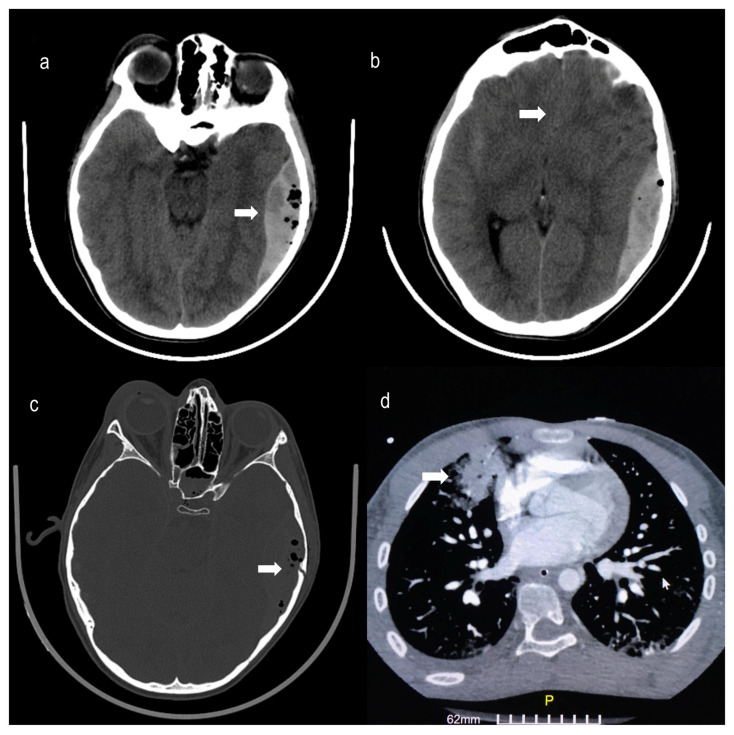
Brain Computed Tomography (CT) scan within 2 h of the accidentshowing: (a) acute left temporal and parietal epidural hematoma (arrow) (5 × 8 cm) with (b) mass effect and 4 mm midline shift (arrow), (c) Bone window of head CT scan showing a complex nondisplaced comminuted fracture of the left temporal bone (arrow), and (d) Chest CT showing contusions of the right upper lobe (arrow), right middle lobe and lower left lobe of the lungs (GE Healthcare Revolution 128-slice CT scanner, USA—Slice thickness of 1.25–5 mm with 3D Reconstructions).
